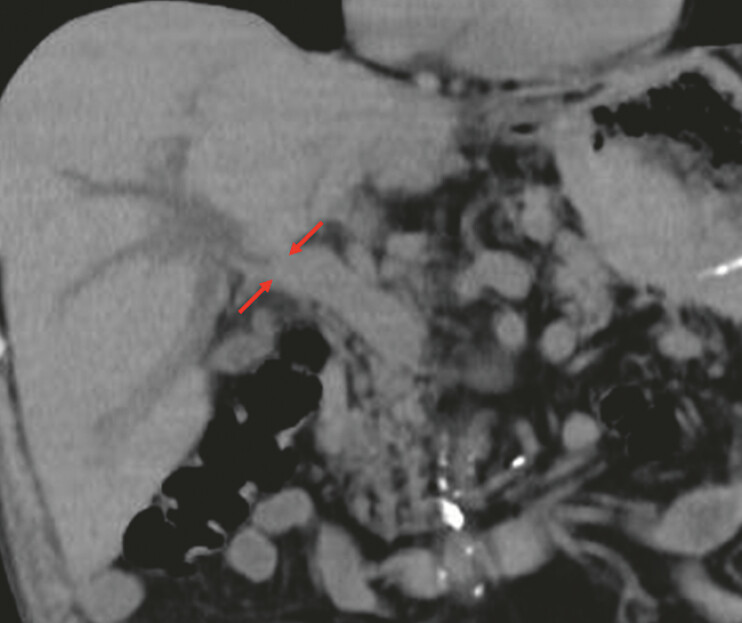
Computed tomography revealing biliary stricture (red arrow) in the hilar bile duct.
